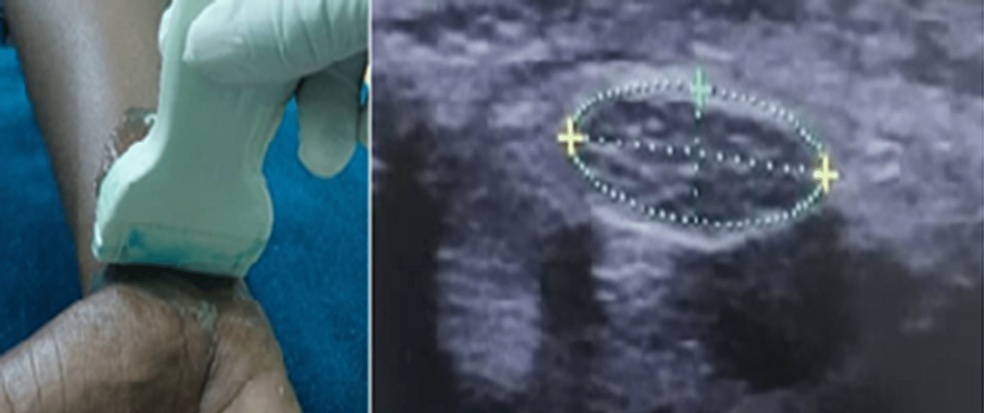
Transverse ultrasound image showing the method of scanning and the measurement of median nerve area in the wrist joint at the carpal tunnel inlet (pisiform level) The photo was taken from the study participants
